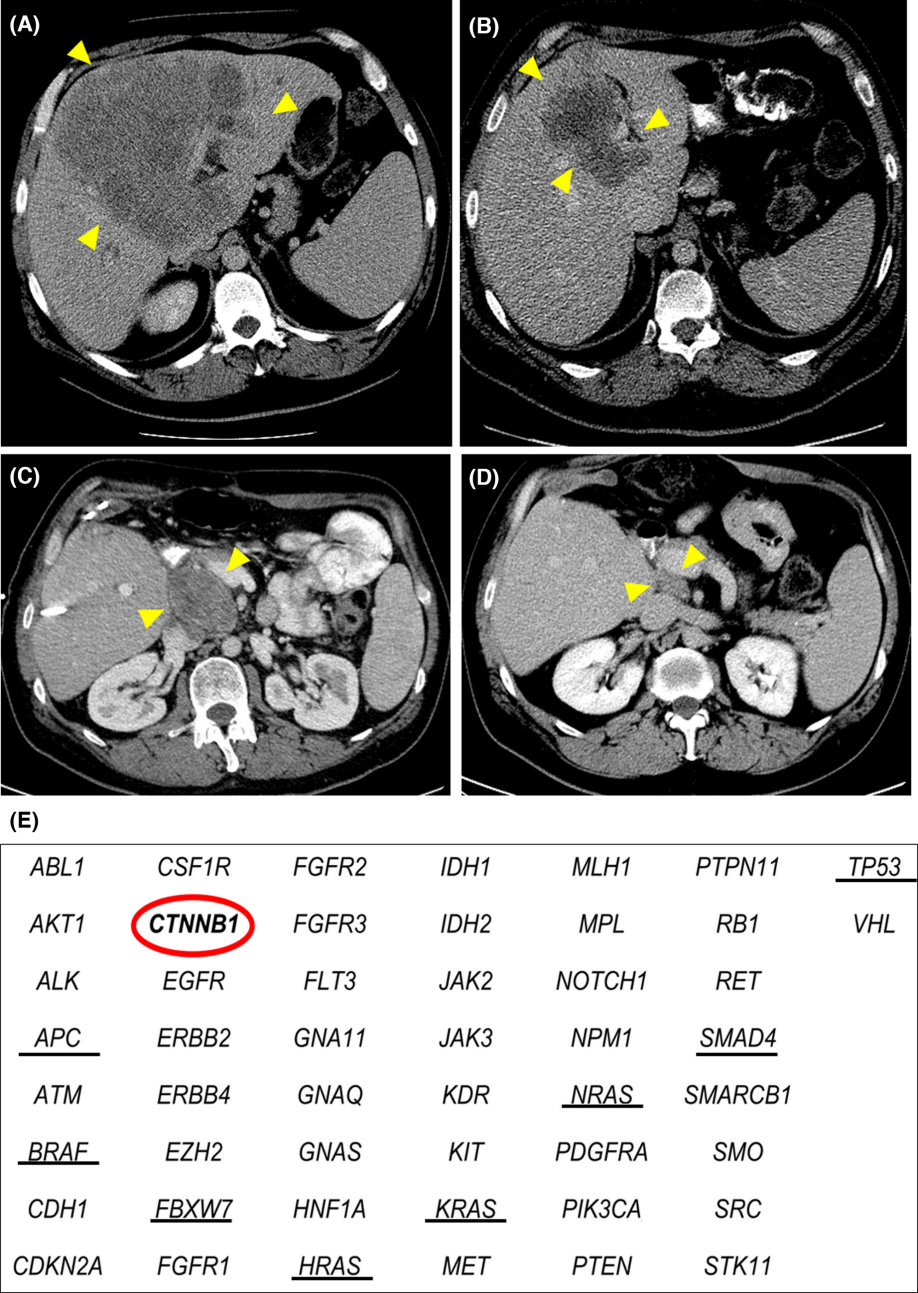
Disease course in a patient who presented with colorectal liver metastases (CLM) centrally located across both hepatic lobes and the caudate lobe after previous resection of primary colon cancer. Yellow arrowheads indicate the tumor. (A) Computed tomography (CT) image at the time of the initial visit showing a large tumor centrally located extending to the hilar plate and caudate lobe. (B) CT image after preoperative chemotherapy showing partial response with persistent invasion of the hilar plate and caudate lobe. (C) CT image 7 mo after extended left hepatectomy with common bile duct and caudate lobe resection showing recurrence in a retro‐portal lymph node. (D) CT image after chemotherapy showing partial response. (E) Results of gene panel analysis of 50 genes. The red circle indicates *CTNNB1* alteration of the tumor, and black underlines indicate driver genes associated with oncologic outcome after resection of CLM. Please note the absence of driver gene alteration in keeping with the good prognosis observed in this patient.
